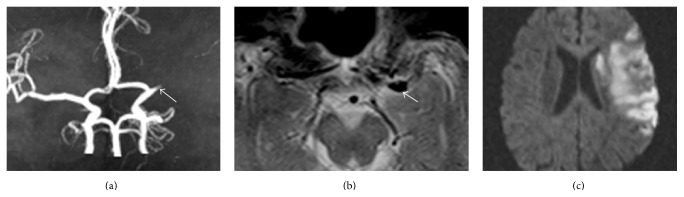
The MCA susceptibility sign in CE patients. (a) MRA reveals an MCA occlusion. (b) T2^*∗*^-weighted MR imaging indicates a large diameter and a short, low-signal image along the MCA. (c) DWI shows a large-acreage cerebral infarction.
